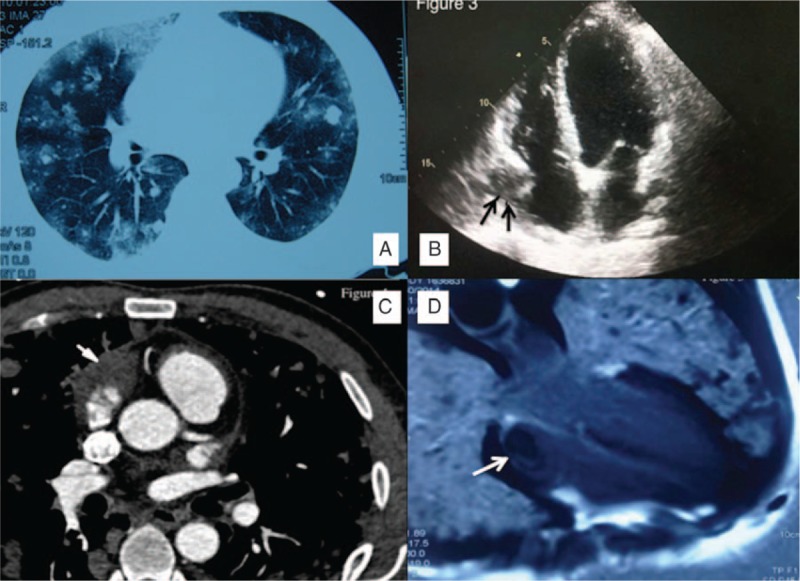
Radiological presentations of pulmonary metastatic angiosarcoma. (A) Chest computed tomography of pulmonary metastatic angiosarcoma. Multiple unequally sized and sharp-margined nodules are seen, mostly distributed in the peripheral regions. Some ground glass opacities are seen, either surrounding the nodules or unassociated with nodules. (B) Echocardiographic manifestation of cardiac angiosarcoma. A mass is seen arising from the lateral wall of the right atrium (black arrows). (C) Appearance of right atrial angiosarcoma on CT angiography. An irregular soft tissue mass is seen in the right atrium (white arrow). (D) Right atrial angiosarcoma on enhanced cardiac MRI. A low signal was found in the right atrium, but the echocardiographic study was negative (white arrow).
